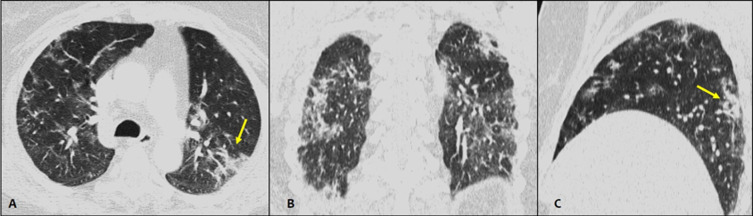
axial (A) coronal (B) and sagittal (C) CT images without intravenous contrast showing bilateral and subpleural pulmonary ground-glass predominant, associated with linear and sub segmental condensation (arrows)
